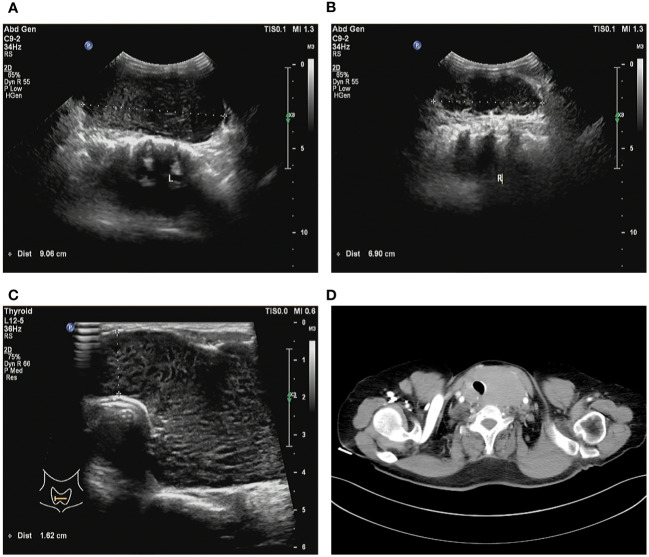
Preoperative imaging images. (A) Ultrasound examination detected the left thyroid gland measuring approximately 9 cm. (B) Ultrasound examination detected the right thyroid gland measuring over 6 cm. (C) The isthmus of the thyroid displayed a thickness of 1.6 cm. (D) Computed tomography imaging demonstrates significant enlargement of the thyroid gland, resulting in evident compression and displacement of the trachea.
